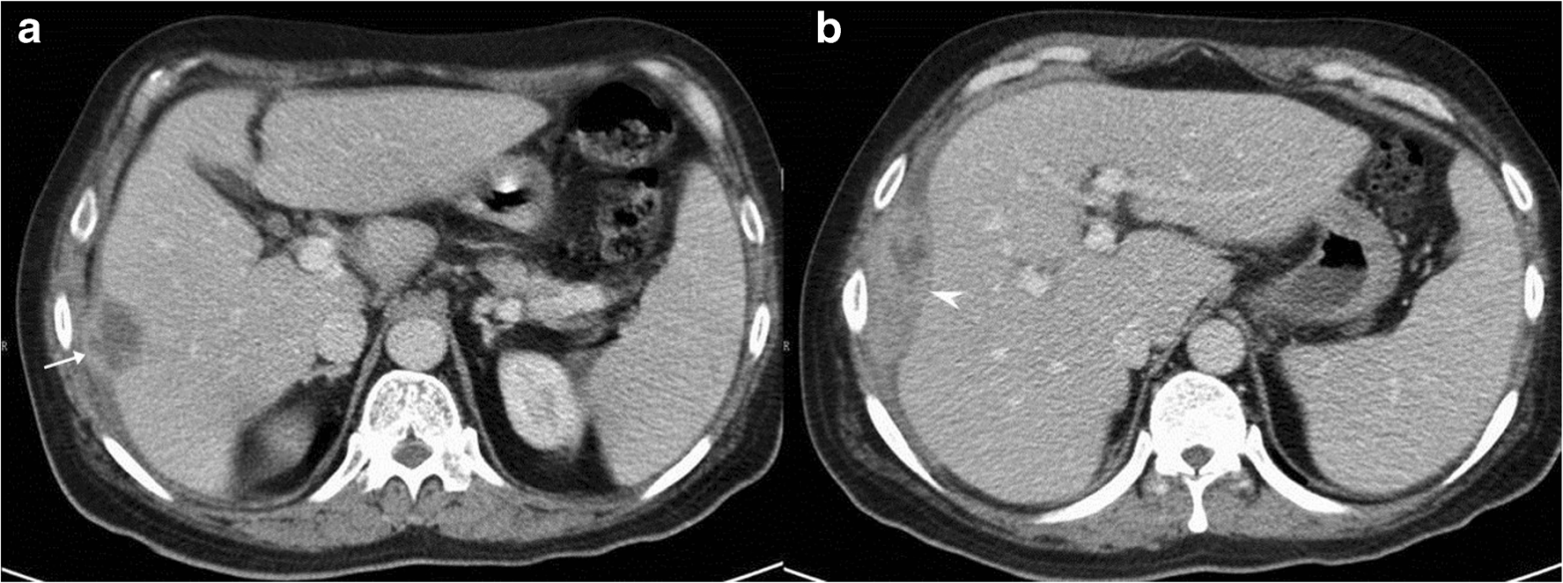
A 45-year-old man with right upper quadrant pain and fever. a, b Axial portal venous phase CT shows a hypodense lesion in the right lobe causing focal contour bulge and capsular thickening (*arrow*) with an associated organised collection in the perihepatic space (*arrowhead*). This was a patient with isolated hepatic tuberculosis with contained rupture of the tubercular liver abscess
